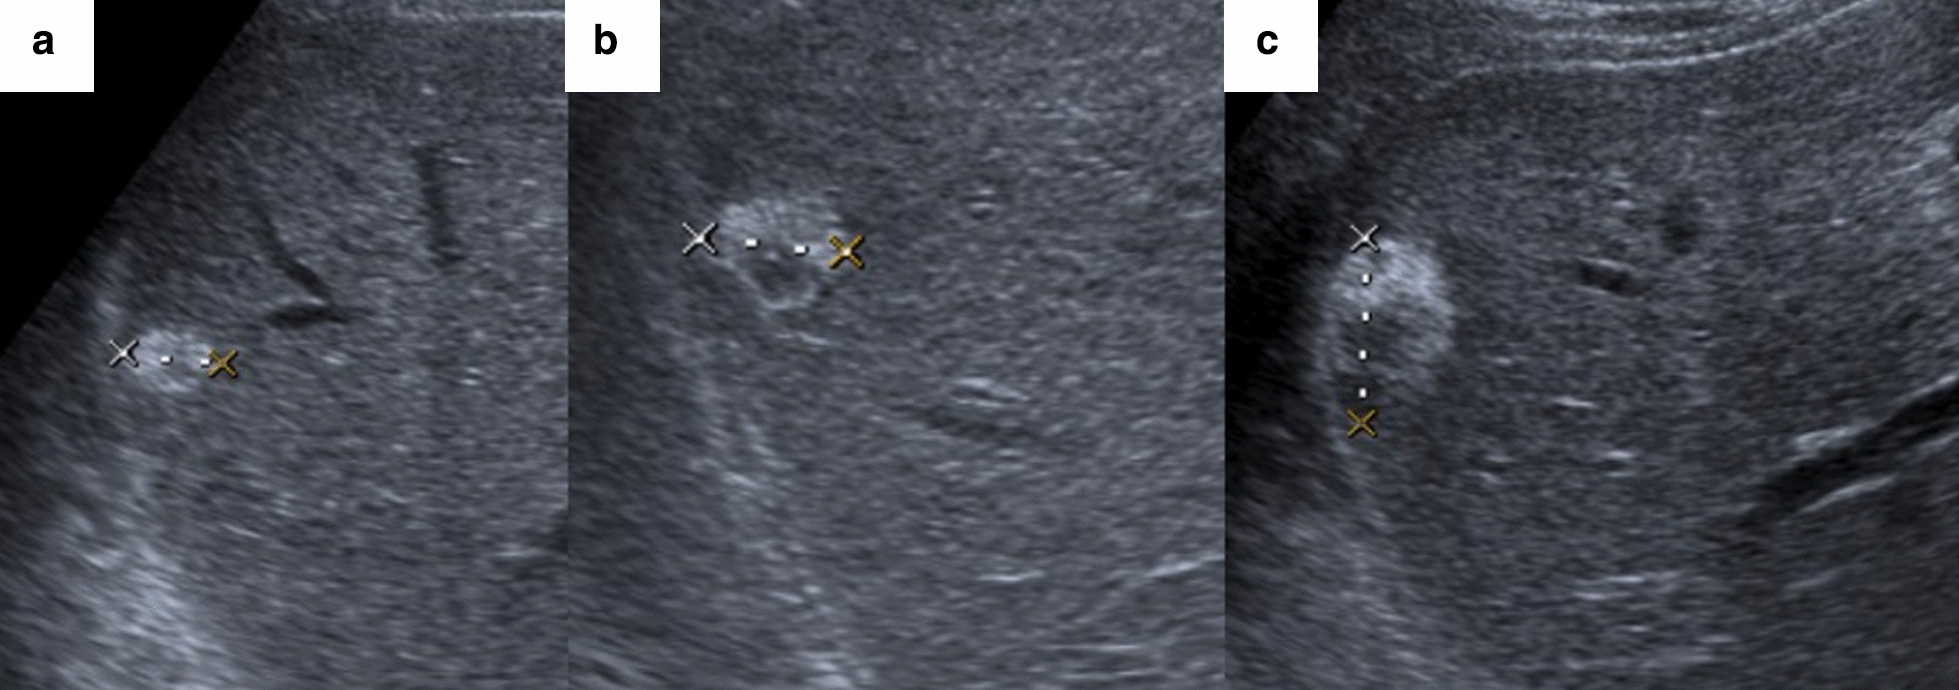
Slowly growing hepatic lesion followed by abdominal ultrasound. A hypercogenic lesion of 13 mm at 2015 (a), and of 19 mm at 2017, with an anechoic zone (b). At 2019 the lesion was heterogeneous, mixed, and its size was 27 mm (c)
